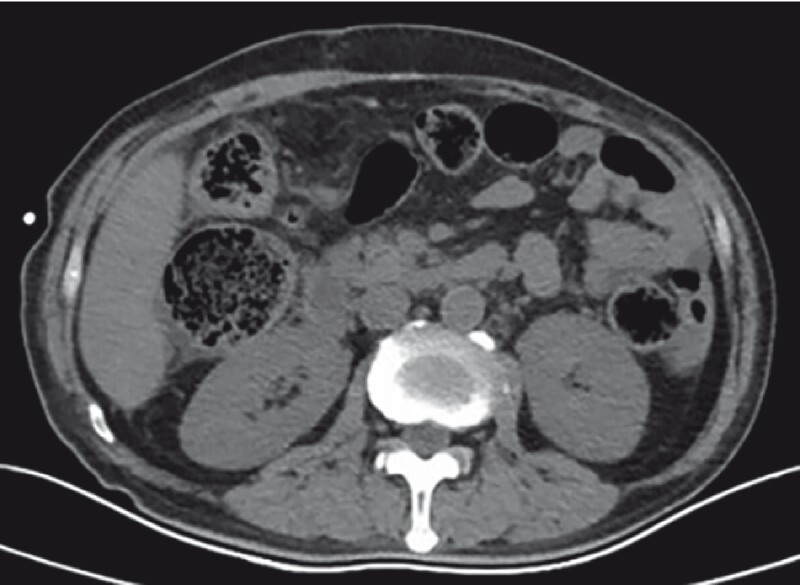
A CT scan taken 53 days after endoscopic treatment, showing the colonic stricture and entire hematoma almost entirely resolved.
